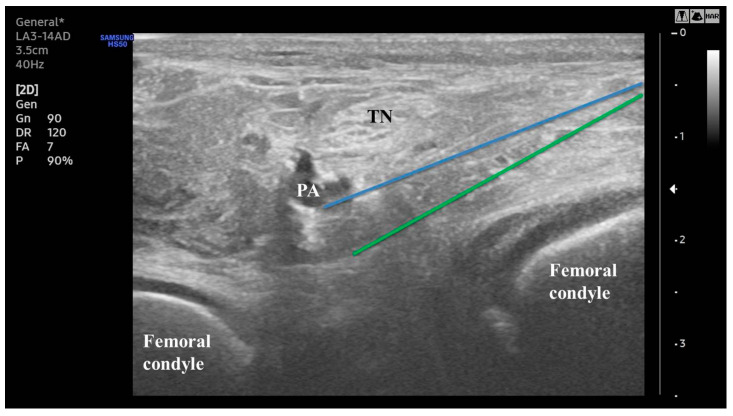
Ultrasound-guided block of iPACK. The blue line represents the needle’s trajectory at point A. The green line represents the needle’s trajectory at point B. PA: popliteal artery; TN: tibial nerve; iPACK: interspace between the PA and the posterior capsule of the knee.
